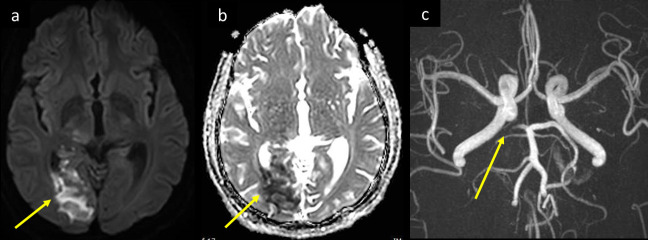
A 58-year-old male diabetic with fever and mild respiratory symptoms was tested positive for COVID-19. He developed confusion, and headache increasing in severity over the past 2 days, associated with dizziness, blurring of vision, and left homonymous hemianopia. MRI (a) axial DWI b 1000, and (b) ADC map shows bright signal and corresponding low signal respectively along the right occipital lobe (arrow in a and b). Intracranial 3D MIP MRA (c) shows thrombotic occlusion of the P2 segment of right posterior cerebral artery (arrow in c). ADC, apparent diffusion coefficient; DWI, diffusion-weighted image; MIP, maximum intensity projection; MRA,MR angiography.
